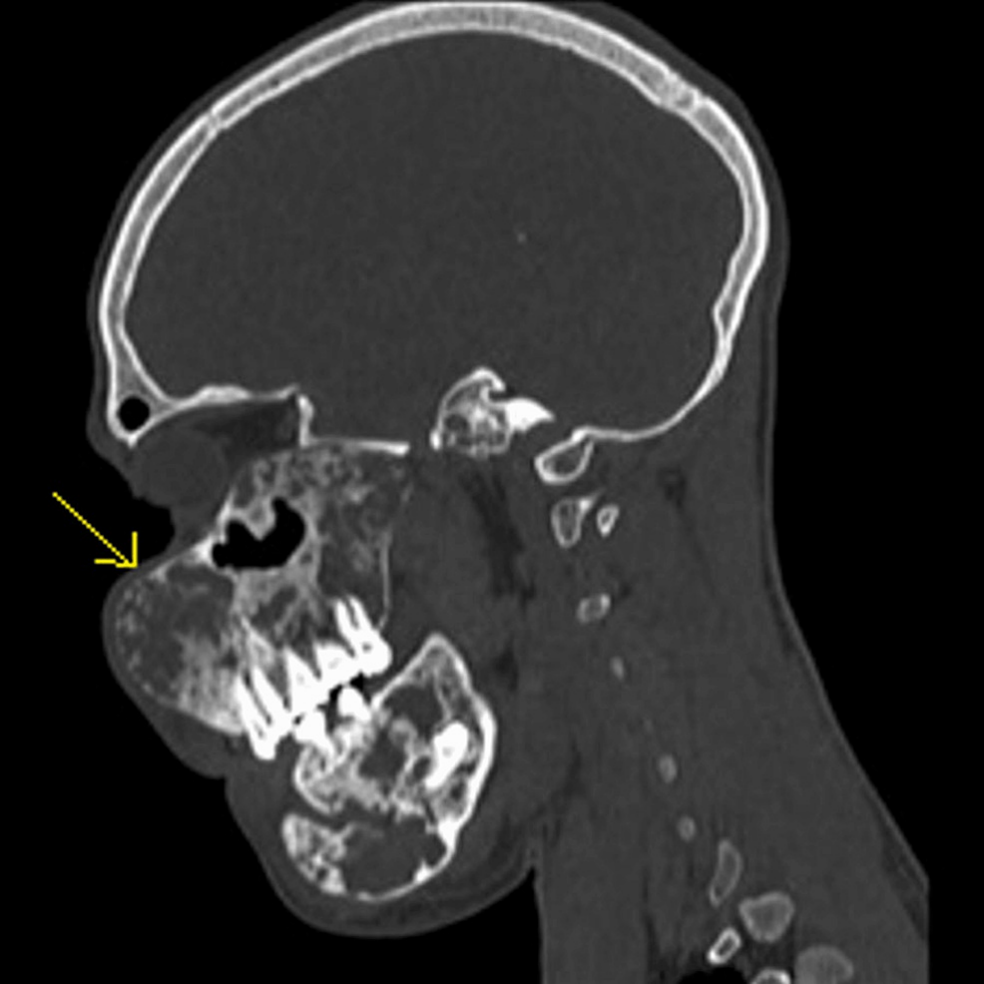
The sagittal section of a reconstructed CT scan of the facial bones reveals an expansile lesion with osteolytic characteristics and a slight ground glass appearance present in both the right upper and lower jaw
